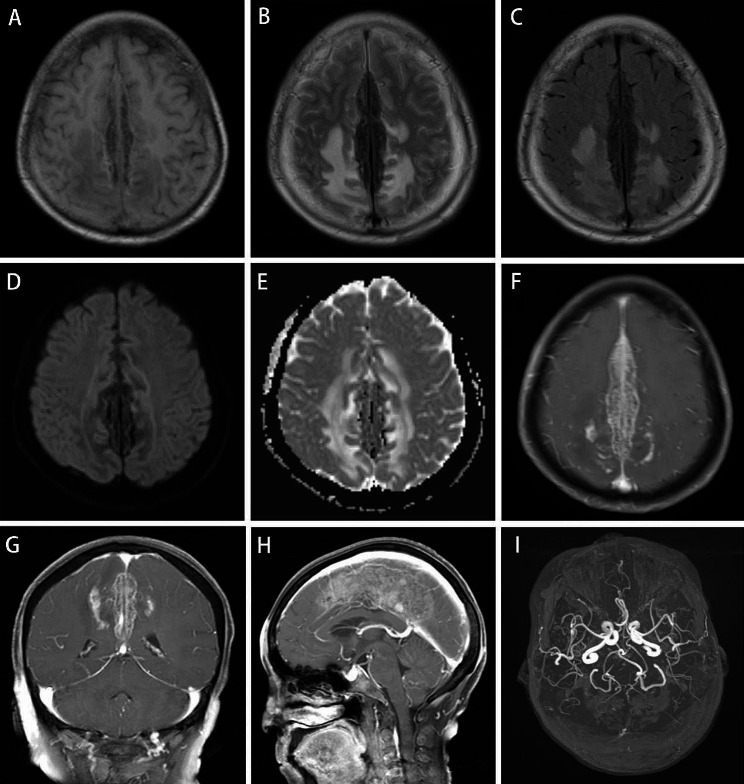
Cerebral MRI of the index patient. The falx of the brain is irregularly fusiform and thickened. Both T1WI (A) and T2WI (B) show low signals. In the FLAIR image (C), large hyperintensity areas can be seen in the bilateral frontal-parietal lobes around the lesion. Diffusion-weighted Iimaging, DWI (D). Apparent diffusion coefficient, ADC (E). The contrast-enhanced scan showed uneven and noticeable enhancement (F). Coronal view (G). Sagittal view (H). No obvious abnormalities were found on MRA of the brain (I)
